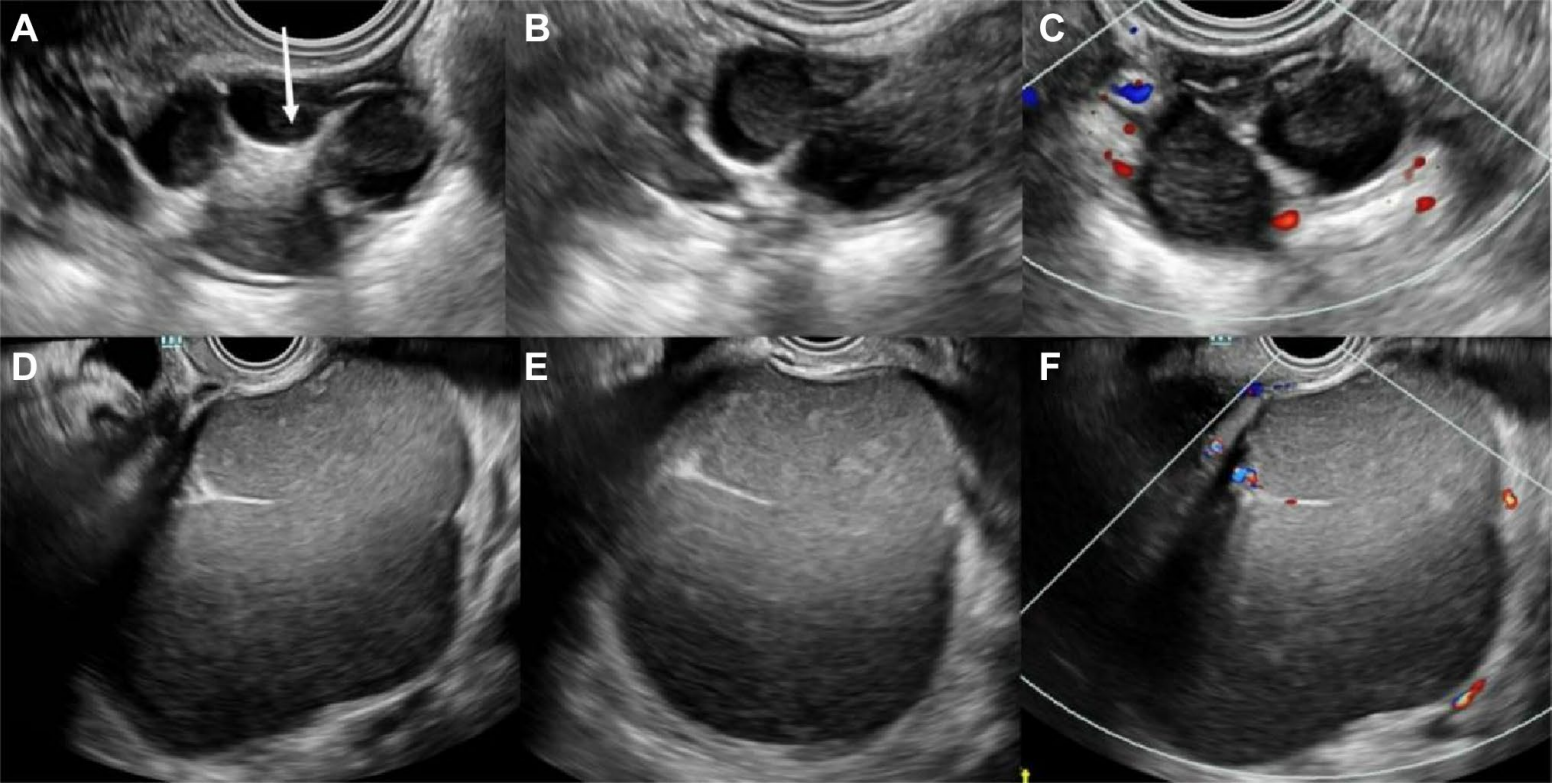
Ultrasound (US) images of classic benign lesions. A–C A 38-year-old woman with a right adnexal lesion pathologically confirmed dermoid cyst. A, B Longitudinal and transverse sections of the lesion. B-mode US showed multilocular cystic lesions, with moderate to high echogenicity in the middle of the lesion (white arrow) and poor sound transmission in the sac. C No clear blood flow signal inside the lesion, and a little peripheral blood flow signal (R1: CS = 1; R2: CS = 2). Senior sonologist (R1) classified lesions as classic benign lesion (O-RADS 2) and junior sonologist (R2) as Multilocular cyst with solid component (O-RADS 4). D–F A 41-year-old woman with a left adnexal lesion pathologically confirmed endometrioma. D, F, Longitudinal and transverse sections of the lesion. B-mode US showed multilocular cystic lesions with ground glass echoes. F Little blood flow signal in the septation (all CS = 2). R1 classified lesions as classic benign lesion (O-RADS 2) and R2 as multilocular cystic lesions (O-RADS 3)
